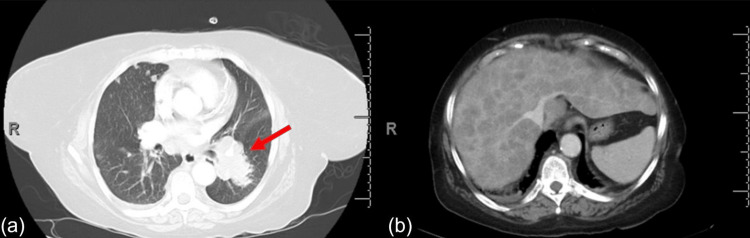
CT of the chest and abdomen demonstrating primary lesion small cell lung carcinoma (a) with metastatic liver lesions (b).
